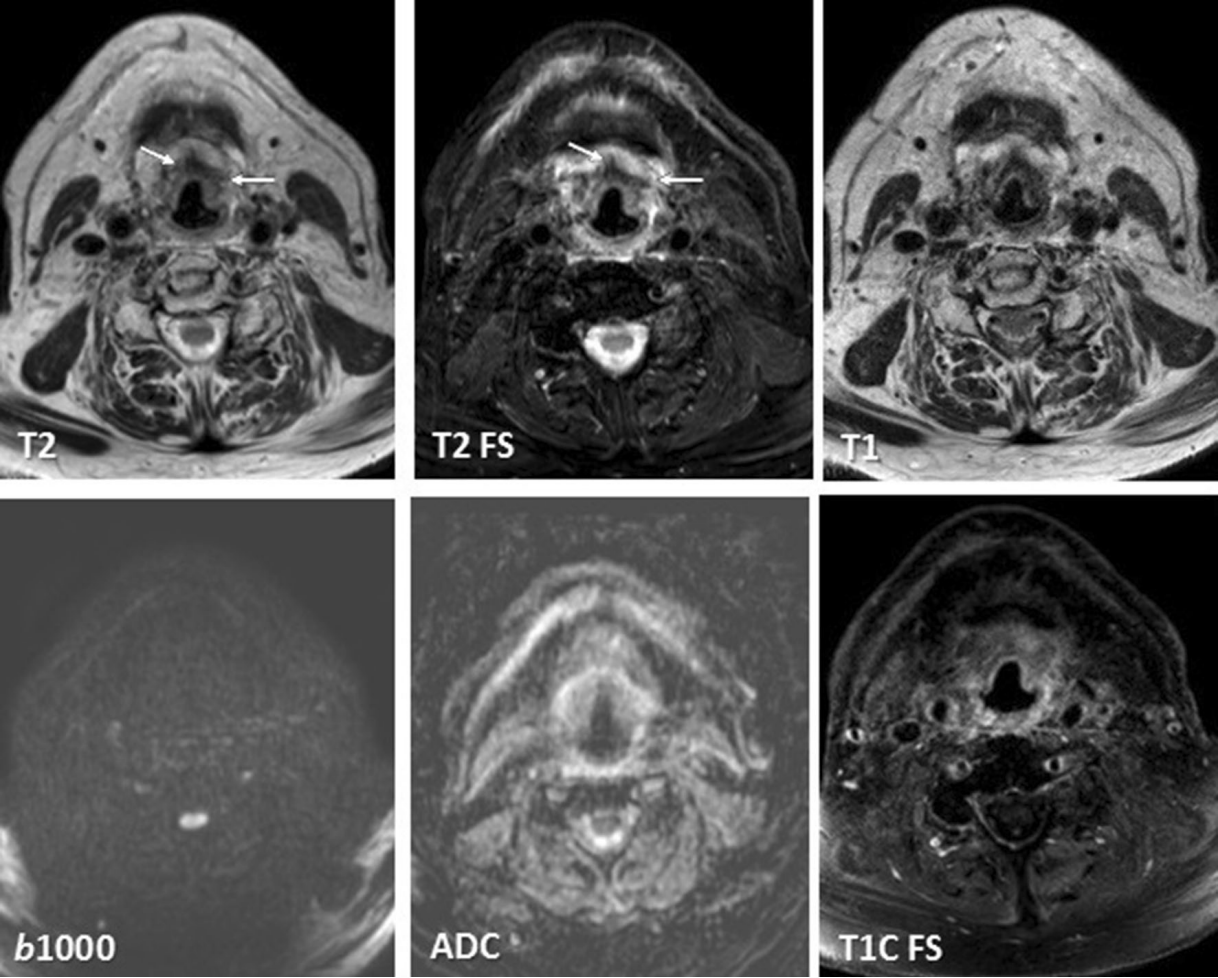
Fibrosis on follow-up MRI confirmed with diffusion. A 67-year-old patient with a T3 vallecula tumor showed fibrosis after radiation therapy with low signal on T1 and T2, no enhancement and no diffusion restriction
